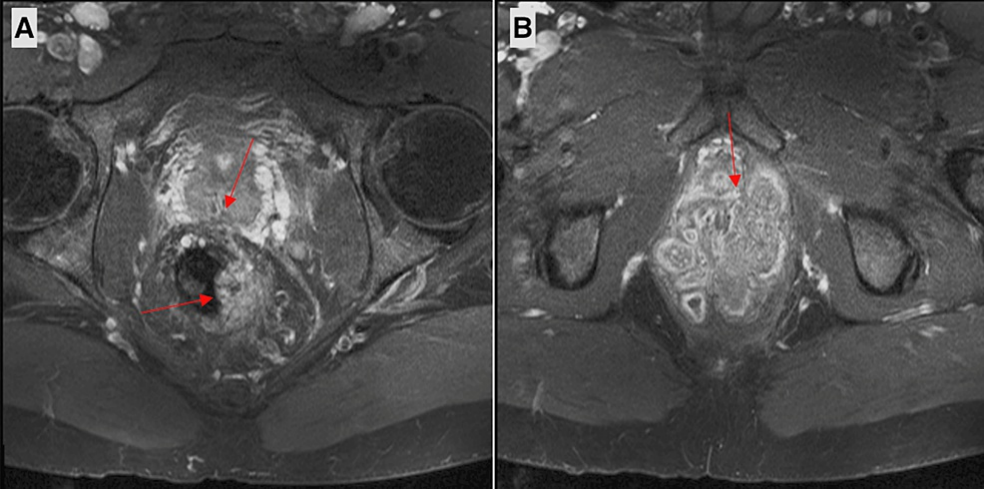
Axial T2-weighted MRI images of the pelvis before radiation therapy showing rectal involvement of the mass at inferior arrow and invasion into the periprostatic space at superior arrow (A) and infiltrating the base of the penis (B).
